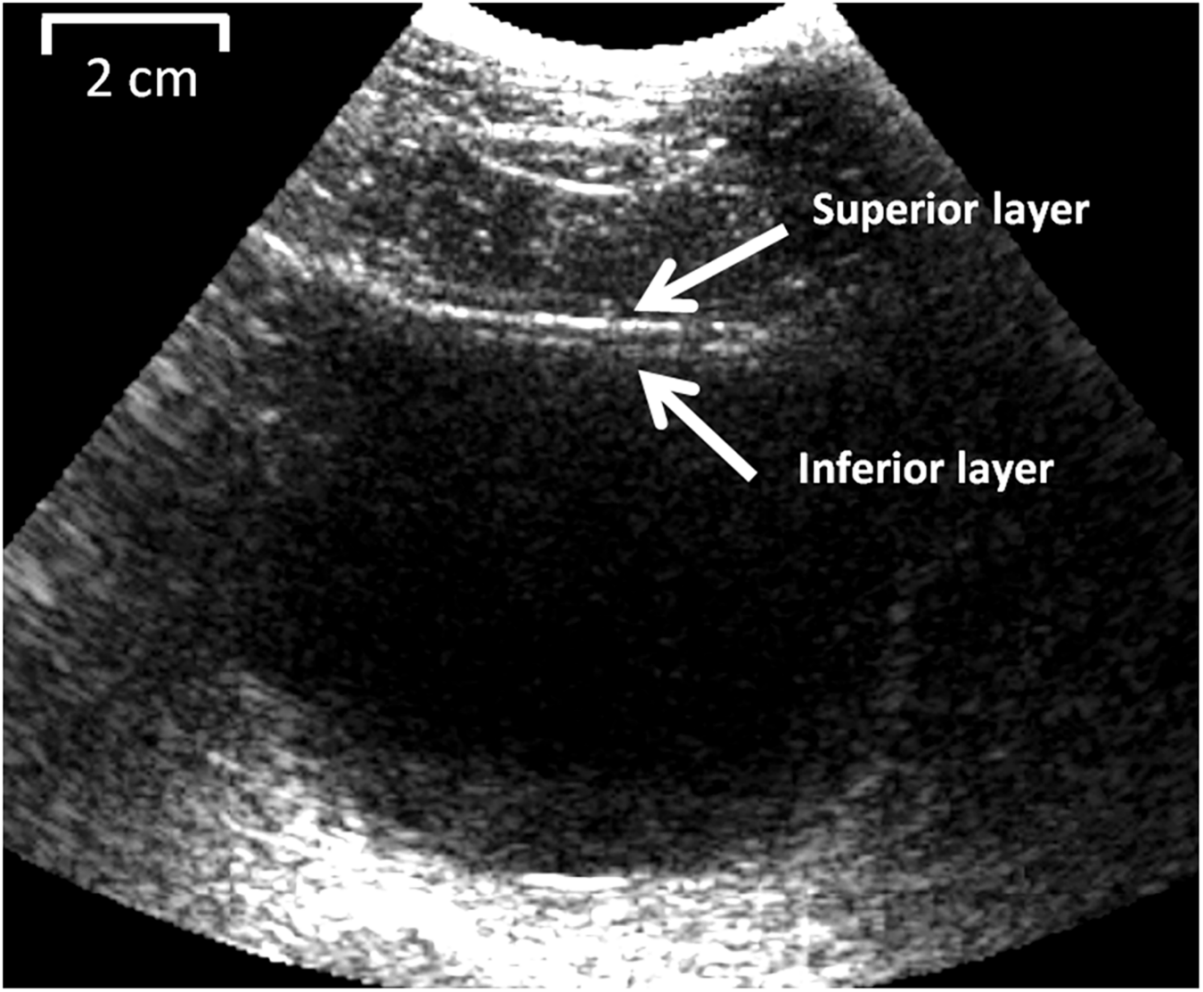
Bladder cavity and detrusor layer (inferior layer) as seen in the B-mode ultrasound image.
